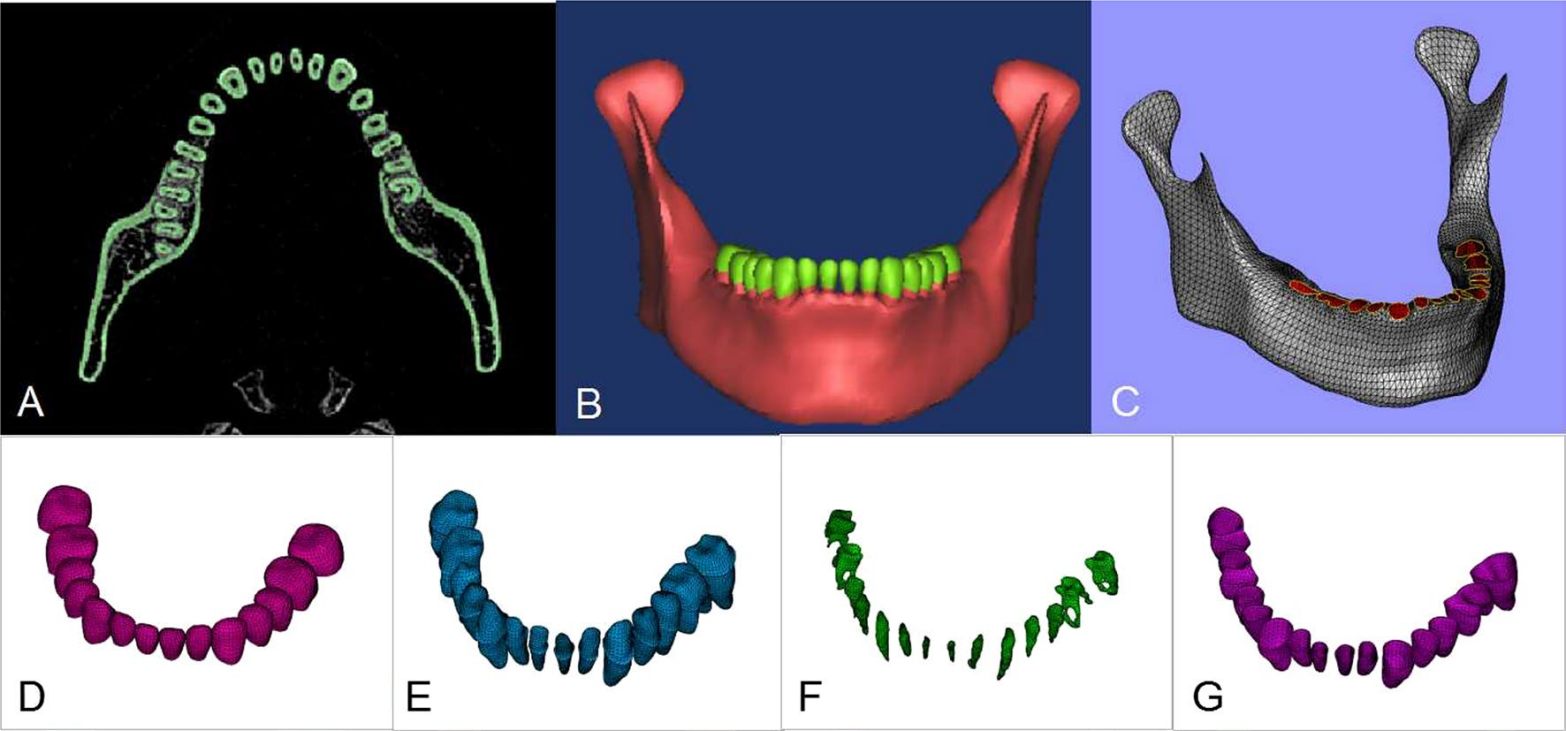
Modeling Steps: A CBCT image of living digital-human No. 23. B the three-dimensional digital anatomy model of mandible. C The mesh refinement of mandible. D The mesh refinement of enamel. E The mesh refinement of dentin-cementum complex. F The mesh refinement of dental pulp. G The mesh refinement of periodontal membrane
